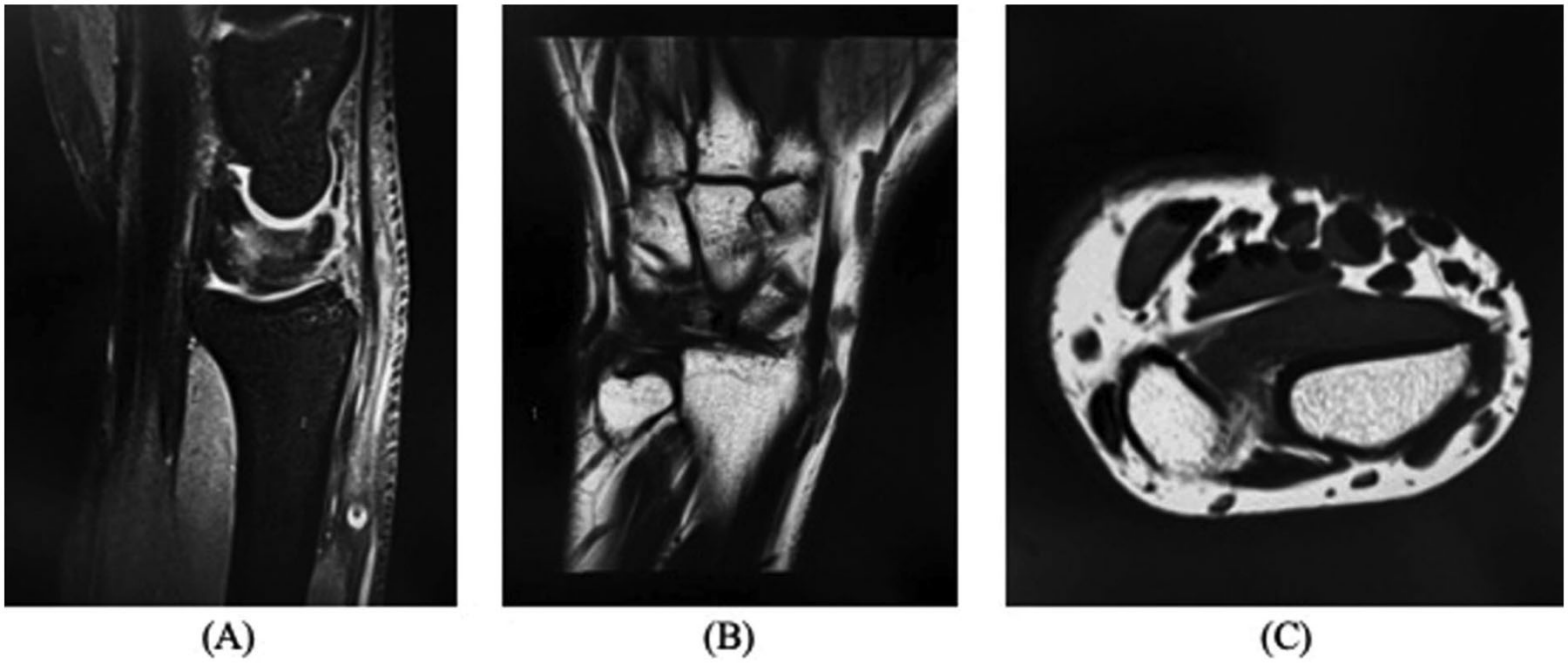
MRI image of a wrist Schwannoma mimicking an intraneural ganglion cyst. A well-defined ovoid structure lateral to the extensor carpi radialis tendon, measuring 6 x 5 mm and communicating with the underlying joint space. (A) Sagittal, (B) Coronal, and (C) Transverse view.
